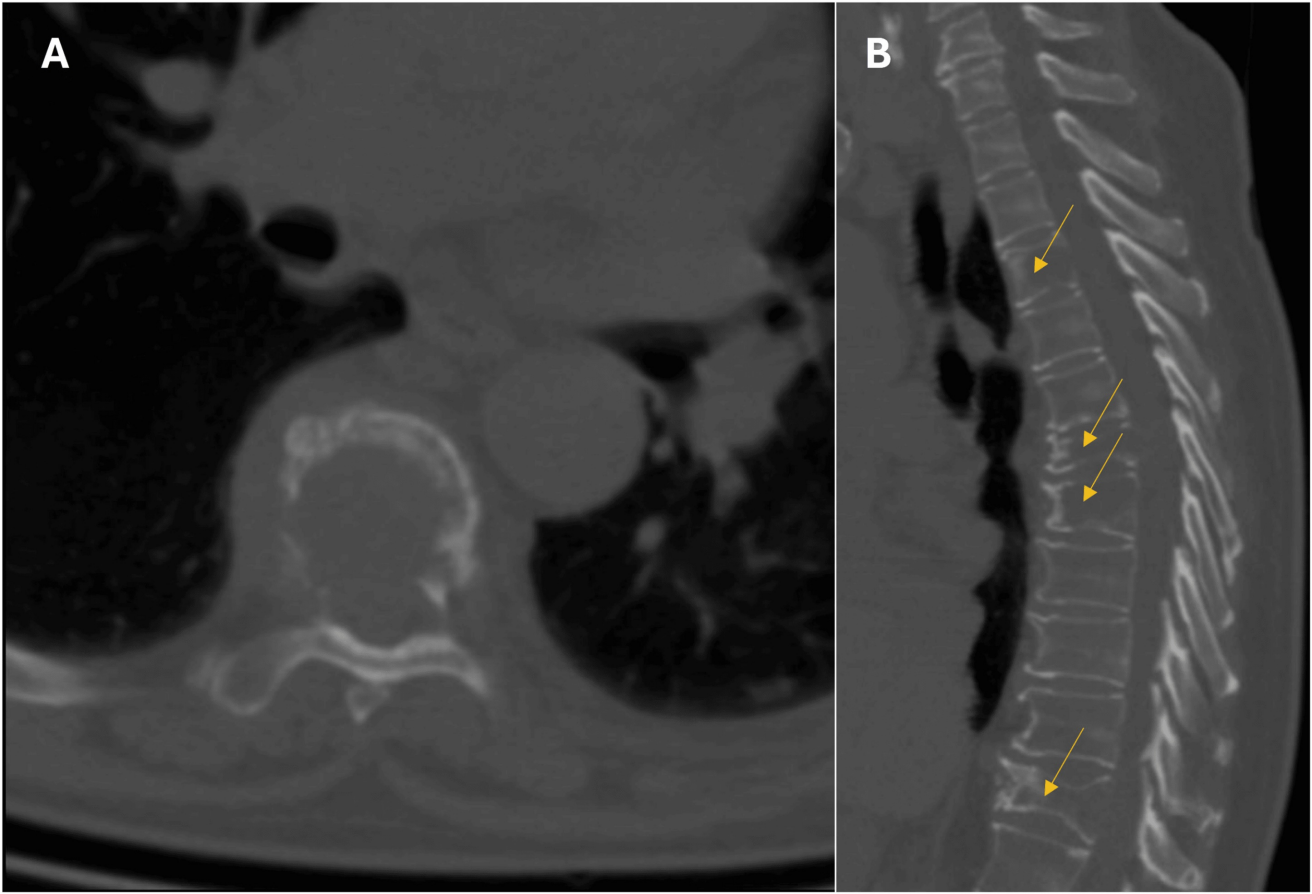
Dorsal spine CT Axial view (A) showing an extensive area of osteolytic process with severe involvement of the vertebral body and the right posterior arch of the sixth dorsal vertebra. Coronal view (B) highlighting multiple areas of radiolucency in the third, sixth, seventh and eleventh dorsal vertebrae suggesting metastatic lesions (yellow arrows).
